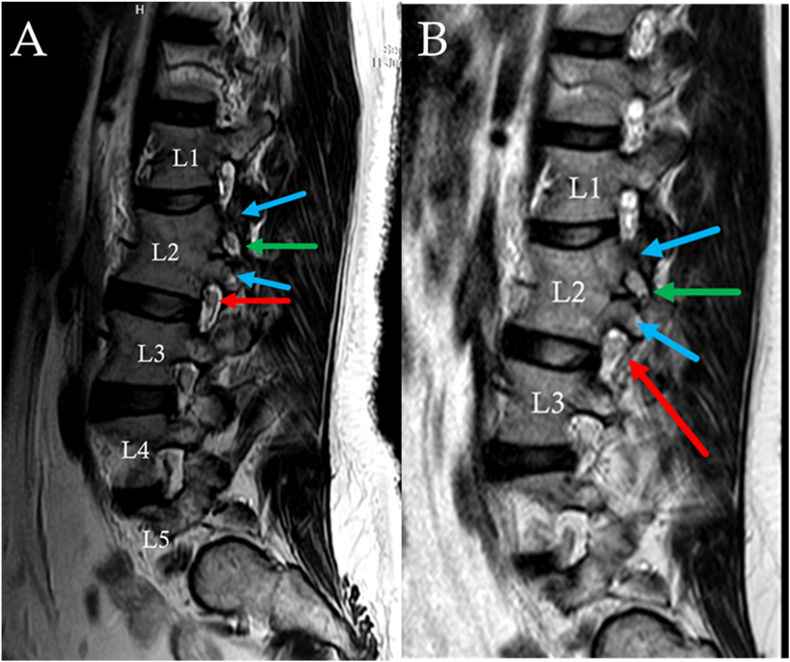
T2-weight MRI images of the lumbar spine. Right-sided sagittal neuroforaminal cuts shown of the patient's 2025 (A) and 2022 (B) studies. Both show duplicate pedicles (blue arrows), typical foramen (red arrow), and “pseudo foramen” (green arrow) containing an exiting nerve root with surrounding moderate neuroforaminal stenosis. (For interpretation of the references to colour in this figure legend, the reader is referred to the Web version of this article.)
